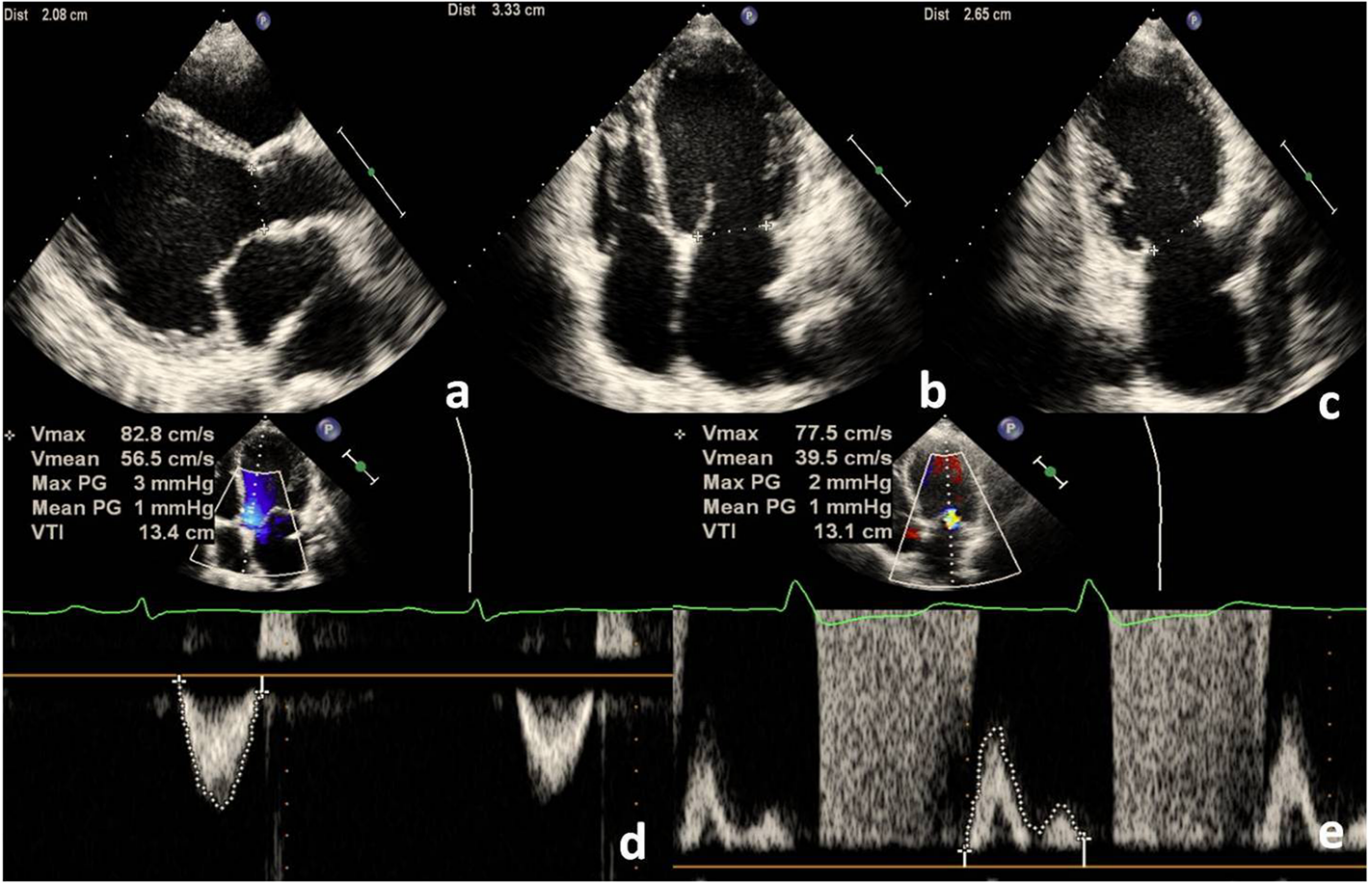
QPD calculations of MRvol assuming that the MA is ellipse geometry. a The diameter of LVOT was measured in the PLAX view, and the CSA_LVOT_ was derived as 0.785 d^2^. d LVOT pulsed Doppler was traced to obtain the VTI_LVOT_. Thus, SV_LVOT_ = 0.785 d^2^ × VTI_LVOT_ = 0.785 × 2.08^2^ × 13.4 = 45.51 ml. b and c The diameter of MA was measured in the A4C and A2C view. The CSA_MA_ was derived as 0.785 × a × b. e MA inflow pulsed Doppler was traced to obtain the VTI_MA._ Thus, SV_MA_ = 0.785 × a × b × VTI_MA_ = 0.785 × 3.33 × 2.65 × 13.1 = 90.75 ml. In this example of MR, MRvol = SV_MA_ - SV_LVOT_ = 90.75–45.51 = 45.24 ml
